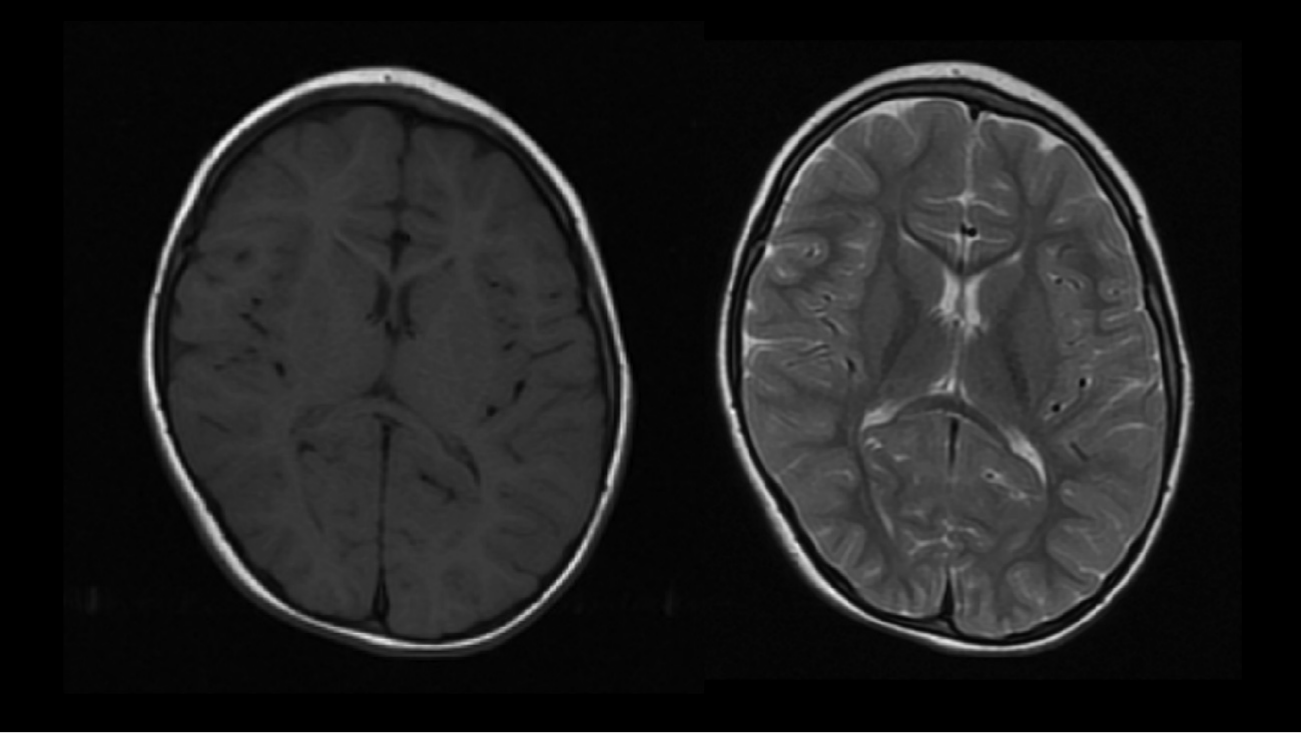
MRI Axial T1/T2 MRI images at 4 years old showed mild atrophy of the frontal cortex and thinning of the corpus callosum. Myelination was complete. No structural abnormalities such as cortical dysplasia were observed.
